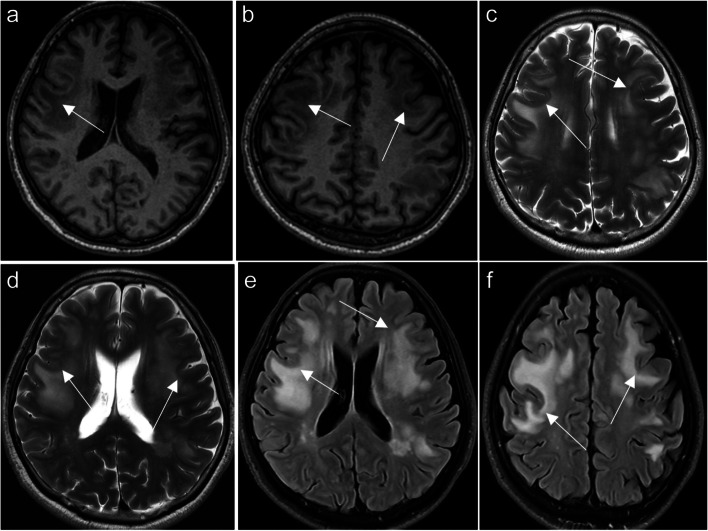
Fludarabine-induced progressive multifocal leukoencephalopathy (PML). A 56-year-old man with chronic lymphocytic leukemia had received a high dose of oral fludarabine 40 mg/m2/d for 5 days every 4 weeks for eight cycles earlier, developed progressive weakness of his left arm and legs. Axial T1-weighted (a, b), T2-weighted (c, d), and FLAIR (e, f). MRI revealed low T1 and high T2 and FLAIR signal intensities in bilateral cerebral white matter particularly involving the subcortical U fibers (arrows) giving a scalloped appearance. These findings are consistent with PML secondary to Fludarabine neurotoxicity
